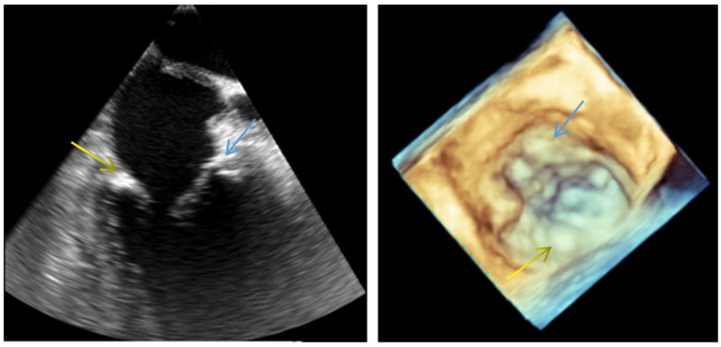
Transesophageal 2D and 3D echocardiography showing severe mitral annulus calcification. Blue arrow: anterior side of the mitral ring; yellow arrow: posterior side of the mitral ring.
